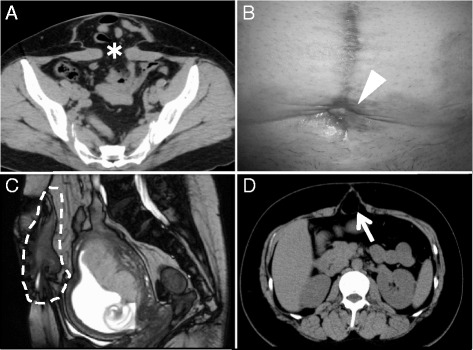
a Lower abdominal computed tomographic scan taken 3 months after the third cesarean section. The *asterisk* indicates abdominal incisional hernia with a 10-cm diameter of the hernia orifice. b Pus was discharged from a cutaneous fistula in the lower abdominal wall. This image was taken at 13 weeks of gestation. The *arrowhead* indicates a cutaneous fistula. c Sagittal, T2-weighted magnetic resonance image of the pelvis taken at 14 weeks of gestation. The *dashed lines* demarcate accumulation of pus surrounding the surgical mesh. d. Upper abdominal computed tomographic scan taken 4 months after the most recent cesarean section. The *arrow* indicates incisional hernia in the upper abdominal wall
